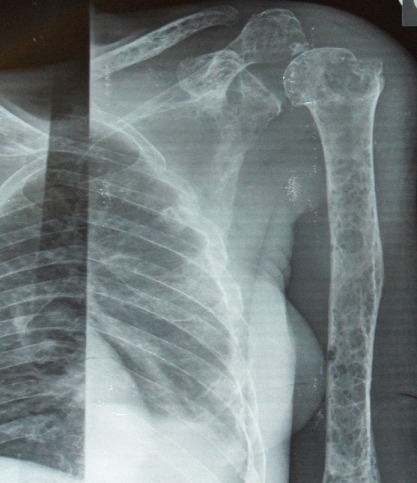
Radiographie de l'humérus gauche mettant en évidence une déminéralisation humérale diffuse, un amincissement des corticales et des géodes à l'emporte-pièce diaphysaire
